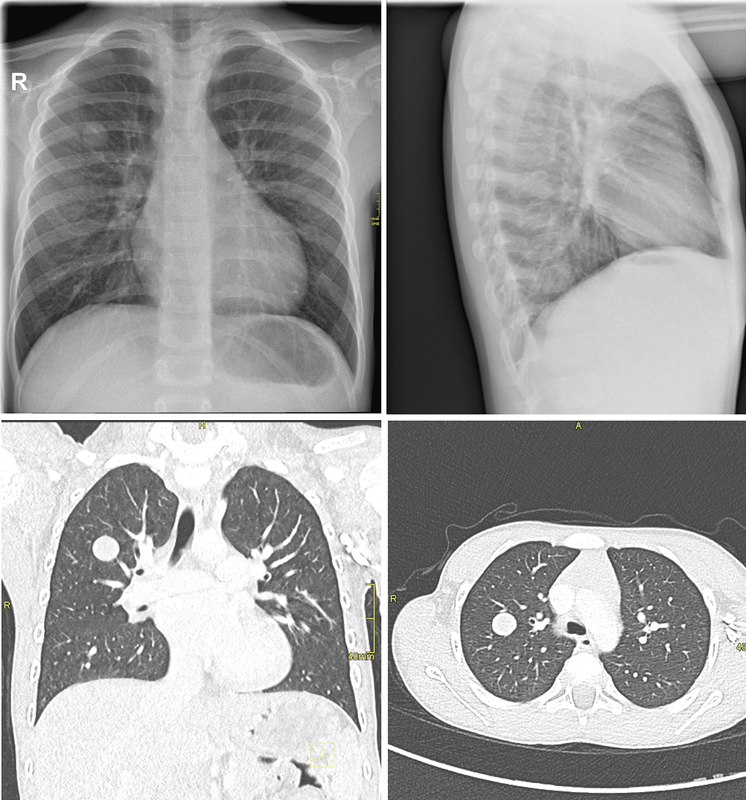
Chest X-ray and computed tomography (CT) scan showing a lesion in the right upper lobe.
